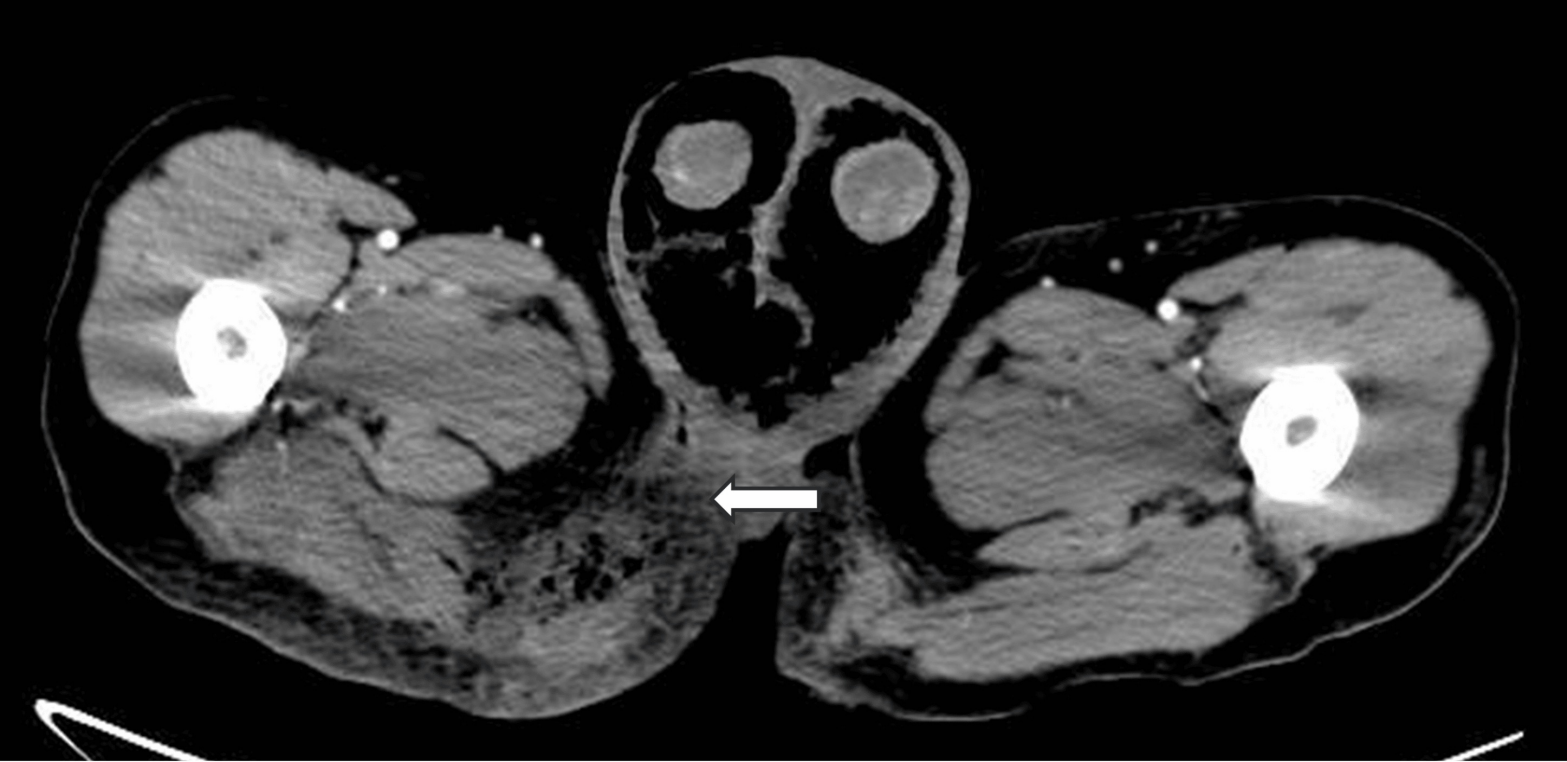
Pelvic CT of the left lower abdominal wall has buttock subcutaneous emphysema.
